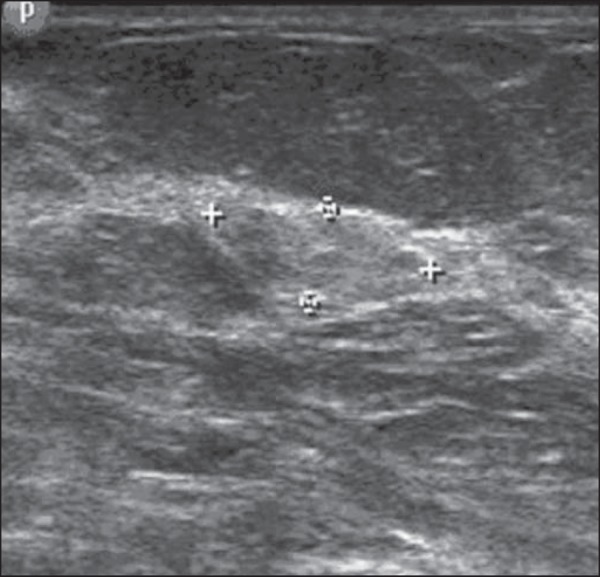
Simple adenosis*. Ultrasonography showing
